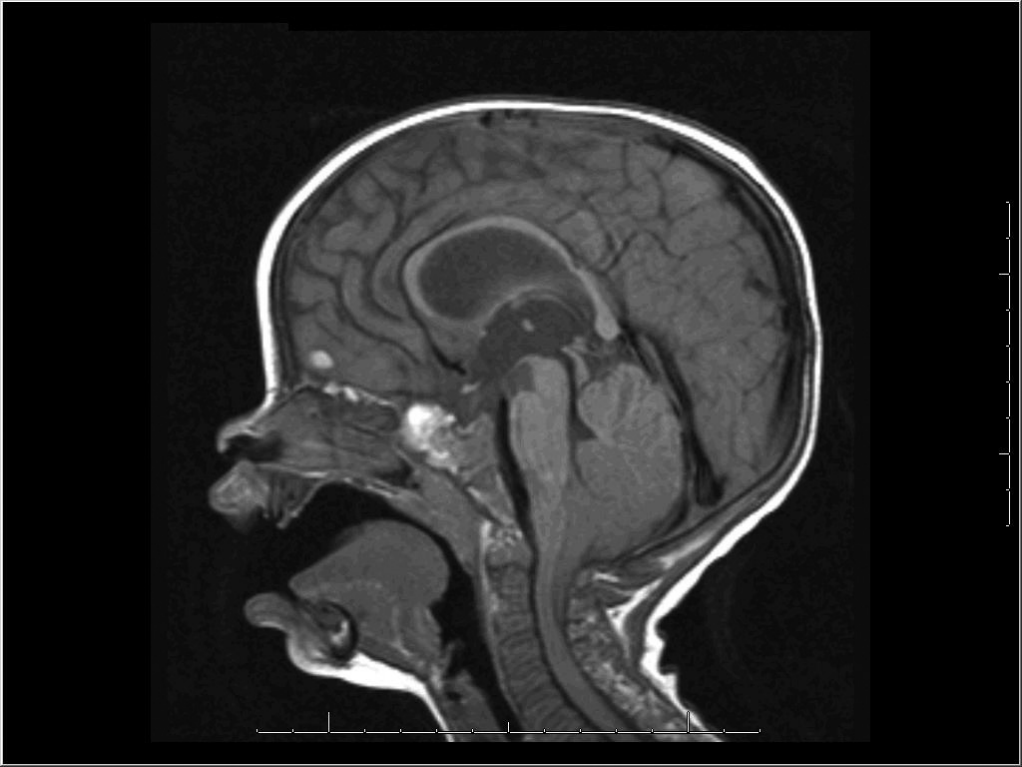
Mid-sagittal T1 weighted MRI of the brain shows tonsillar herniation to the level of C3. There is effacement of the fourth ventricle and Triventricular hydrocephalus.
